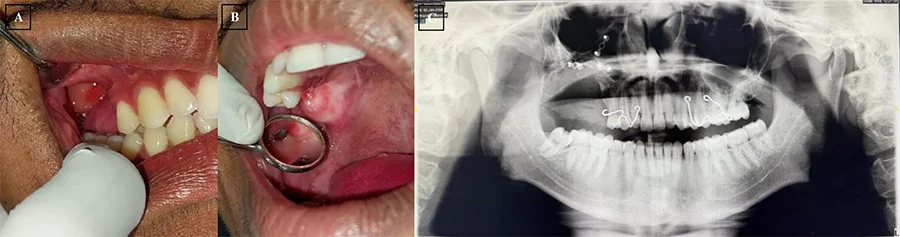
Postoperative follow-up at 16 months: (A-B) Intraoral examination; (C) Panoramic radiography.
